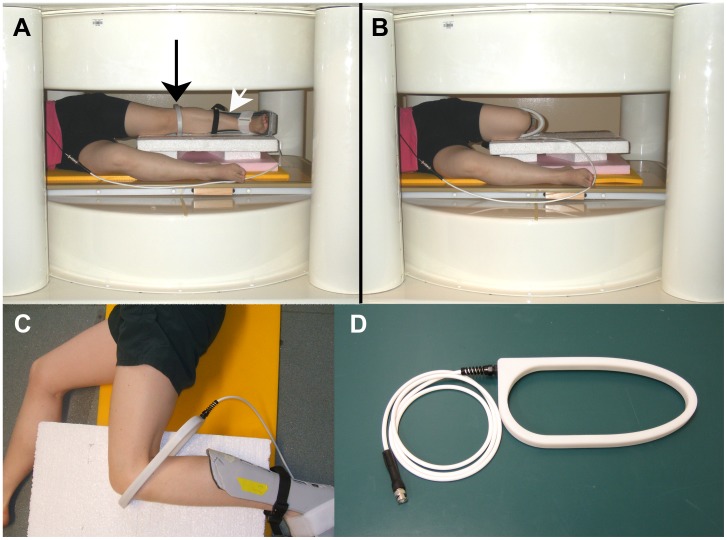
The open-bore MRI scanner and the radio frequency (RF) coil. A subject lying on her side in the scanner wearing the RF coil (black arrow) and foot brace (white arrow), with her knee in relaxed extension (A). Same subject with her knee in flexion (B). An aerial view of the RF coil around a flexed knee (C). The RF coil (D).
